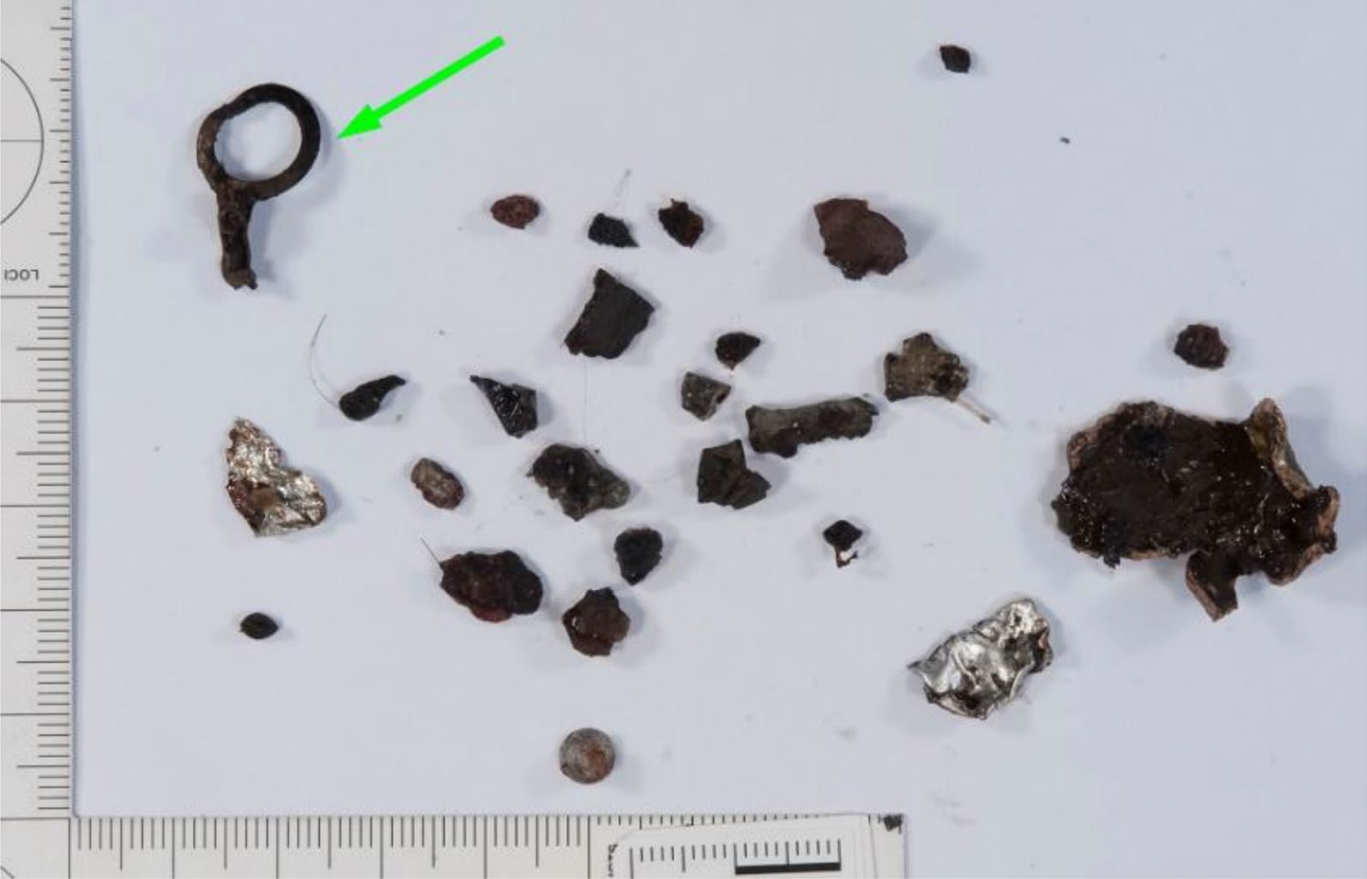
Photograph showing some of the foreign objects recovered from the remains of flight crew member #1 before cleaning. The ring-shaped object (green arrow) is also visible in the CT topogram (Figure 2). Scale: mm.
